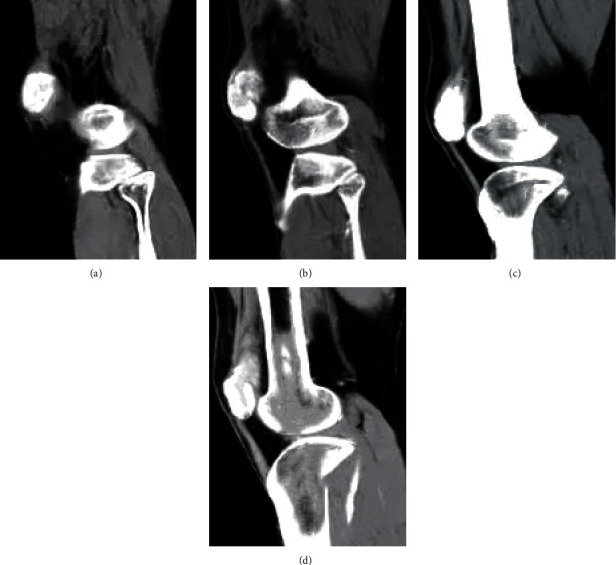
CT images of KOA patients. (a) was of the patient 1, male, 37 years old; (b) was of the patient 2, male, 45 years old; (c) was of the patient 3, male, 42 years old; and (d) was of the patient 4, male, 43 years old.
